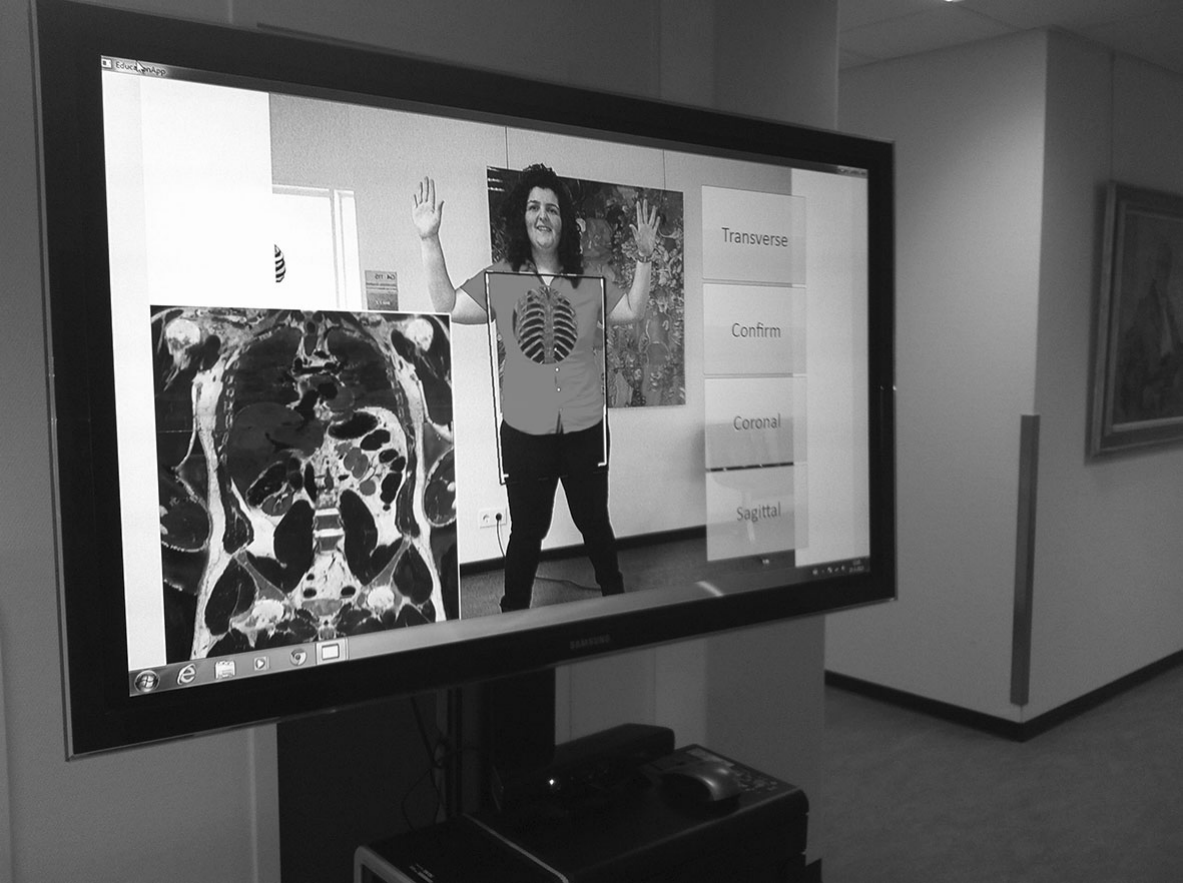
A screenshot of the magic mirror ‘Miracle’ that augments CT data onto the body of a trainee
